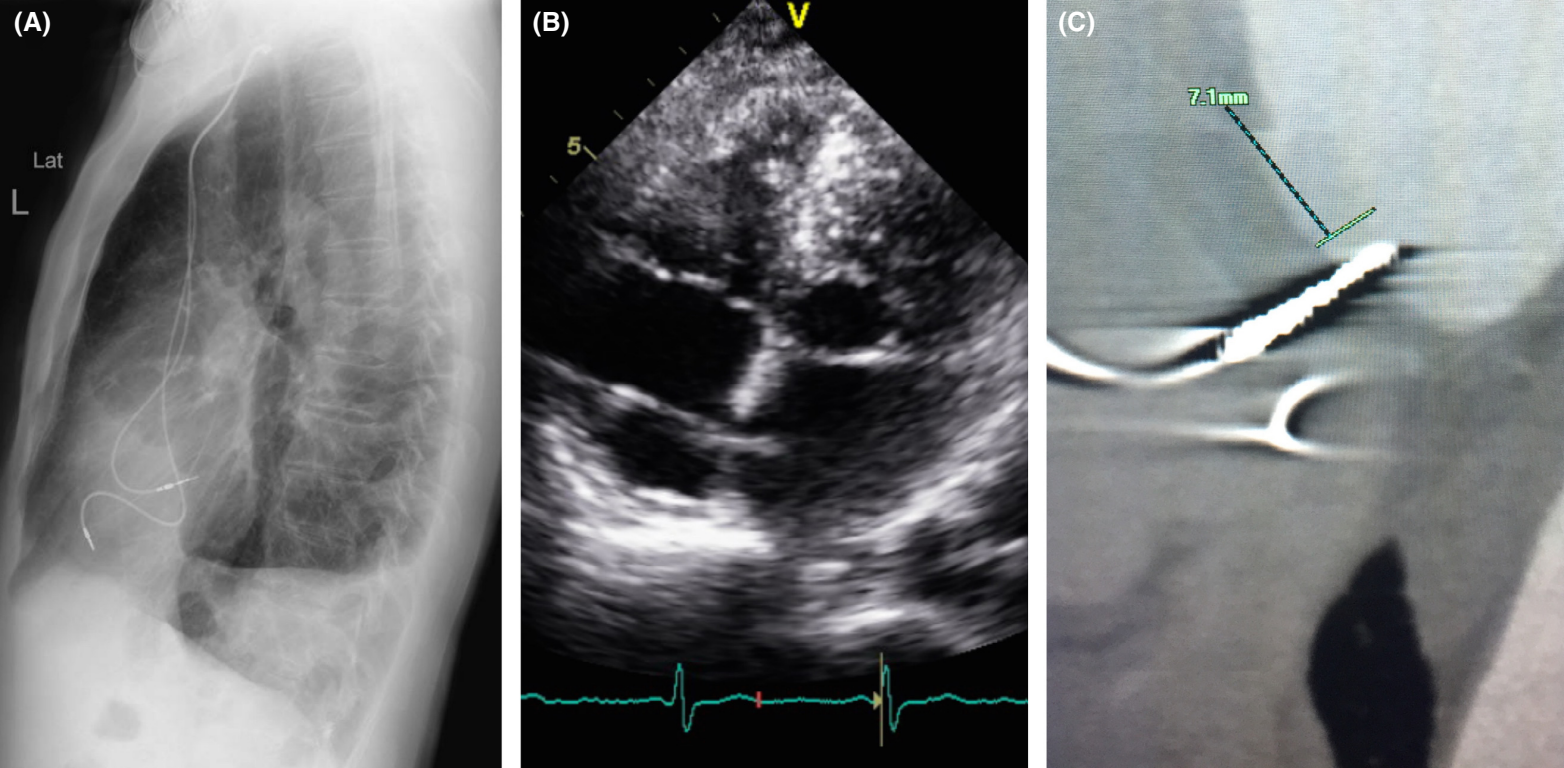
Previous lateral chest X‐ray demonstrating that the atrial lead was more posterior in position that expected for an RA lead (A). Transthoracic echo subcostal view demonstrating lead crossing the intra‐atrial septum to the LA (B). Cardiac CT scan with reported 7.1 mm of the lead is protruding into the left atrium (C).
